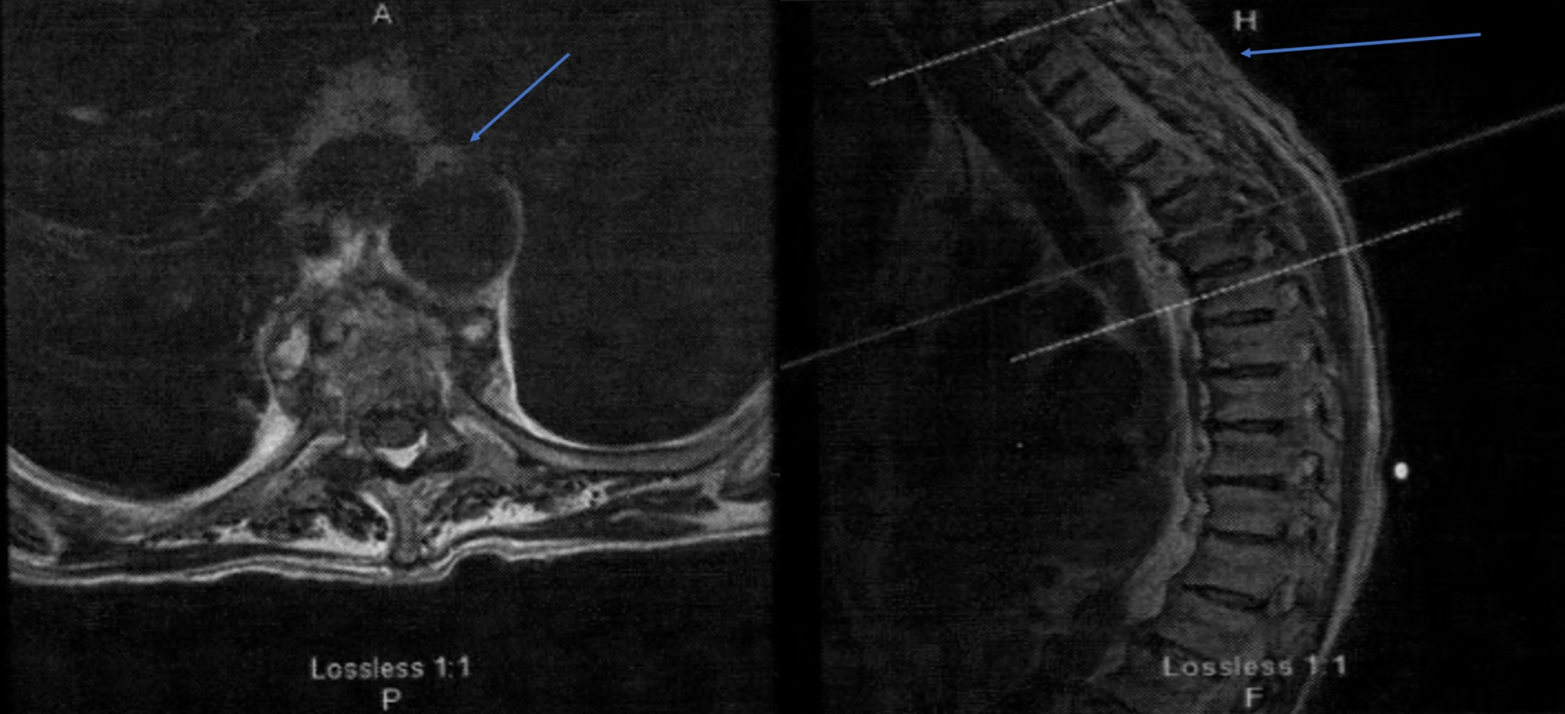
MRI thoracic spine MRI of the thoracic spine showing discitis/osteomyelitis at T4-5 (left) resulting in some elevation of the posterior longitudinal ligament and mild central canal stenosis (right). There was surrounding soft tissue inflammation present anterior and lateral to the spine (left). There was no epidural abscess demonstrated at the time of the exam. The blue arrows demonstrate the findings discussed above
